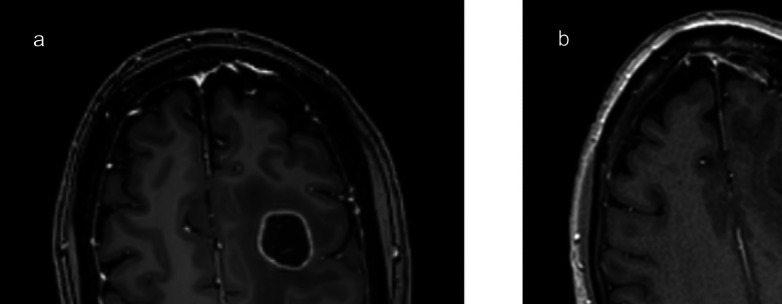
Case 3 – Preoperative MRI (axial view) demonstrated a metastasis in the left middle frontal gyrus (a). Post-operative MRI confirmed a complete resection (b)
